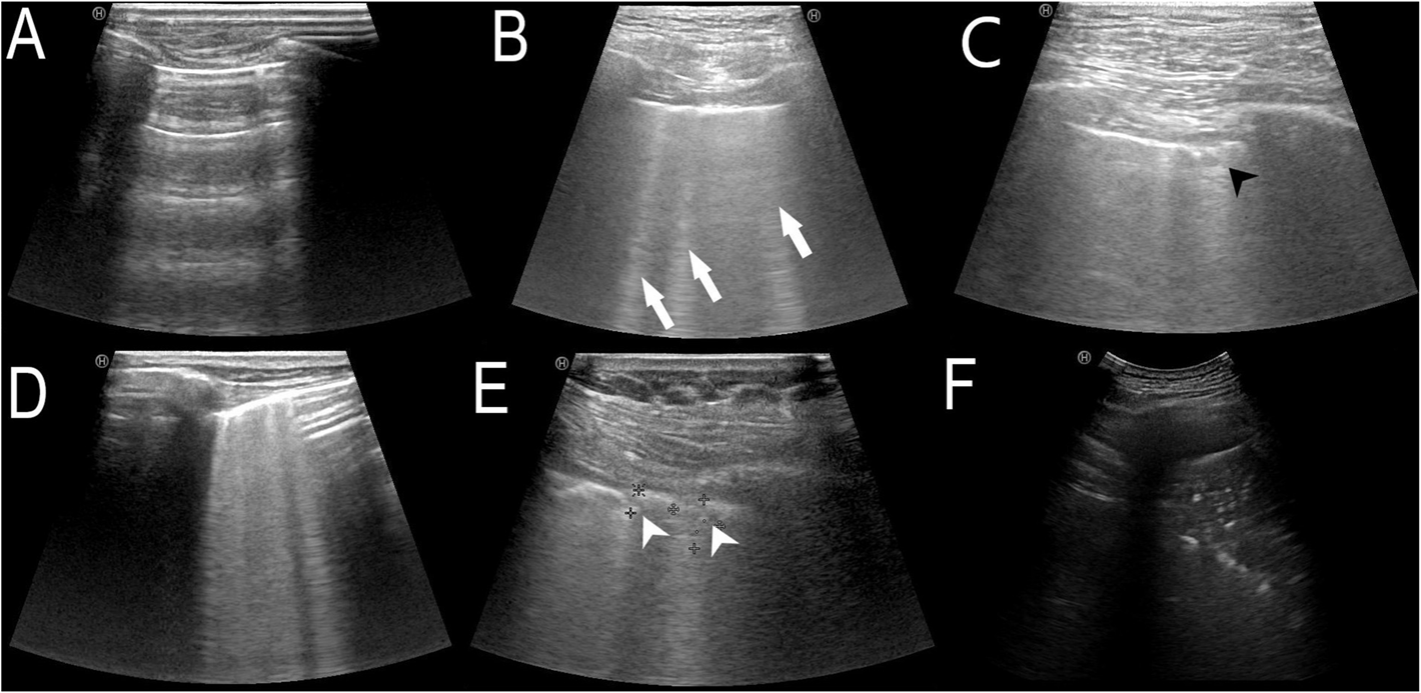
Typical ultrasound patterns with different scores in parentheses. a: A-profile (0 point); b: typical B-profile (1 point). B-lines marked with white arrows; c: small subpleural consolidation (black arrowhead) with clear pleural line (1 point); d: confluent B-profile (2 points); e: multiple subpleural consolidations (white arrowheads) and irregular pleural line (2 points); f: consolidated lung with aerobronchograms (3 points)
